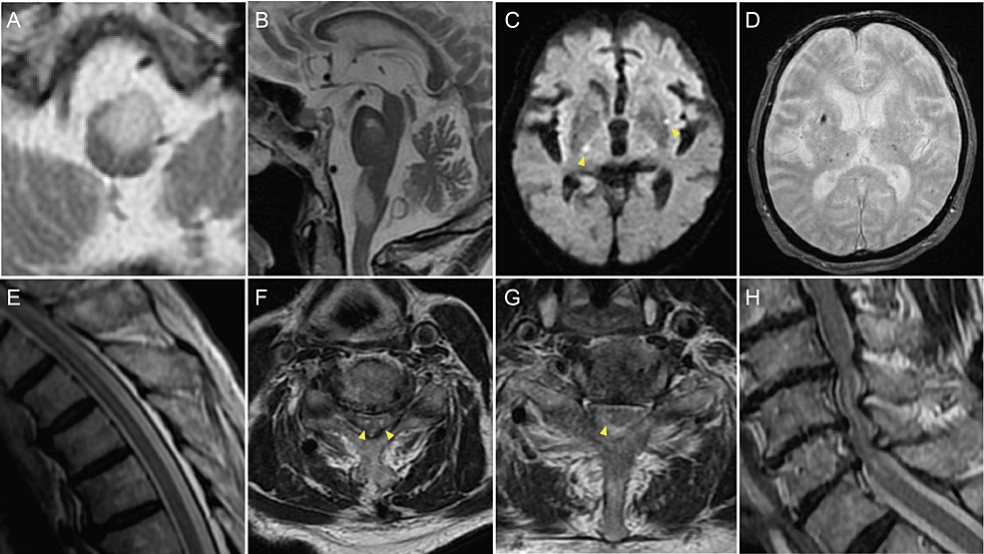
MRI. (A, B) Axial and sagittal T2 images showing massive lesion in the medulla oblongata. (C) Axial diffusion images showing multiple hyperintensity lesions (arrowheads). (D) Axial T2* images showing multiple low-density lesions. (E) Sagittal T2 images showing longitudinal hyperintensity of lateral funiculus from C2 to S5. (F, G) Axial T2 images showing hyperintensity of bilateral lateral funiculi from C6 to T1 and of right lateral funiculi from C2 to C6 and T1 to T7 (arrowheads). (H) Sagittal T2 images showing spinal canal stenosis and hyperintensity area at the level of C5/6 and C6/7.
